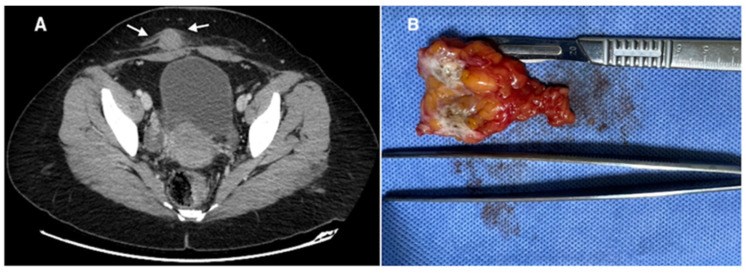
Abdominal wall endometriosis in a 34-year-old woman with a history of two cesarean sections, endometrioma excision, inguinal hernia repair, and appendectomy, presenting with cyclical pain, dysmenorrhea, and palpable swelling along a Pfannenstiel incision scar. (A) Axial computed tomography (portal venous phase) image reveals linear perilesional infiltration (white arrows); (B) macroscopic appearance of the excised endometriotic nodule.
